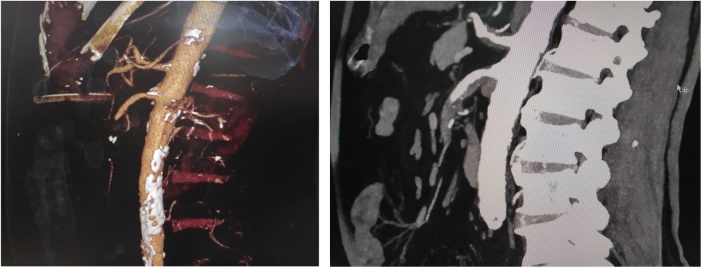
Computed tomography with intravenous contrast showing SMA occlusion.
